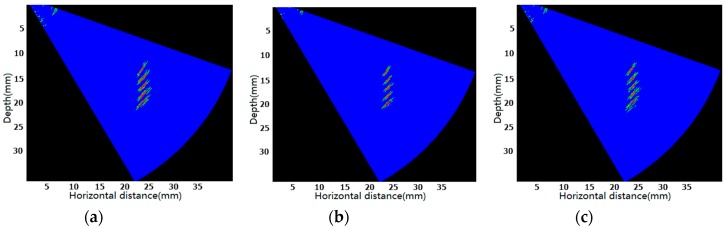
The results of ultrasonic phased array sector scan with different time delay accuracies: (a) 2 ns (4× interpolation CIC filter); (b) 1 ns (8× interpolation CIC filter); (c) 1 ns (8× interpolation half-band filter).
